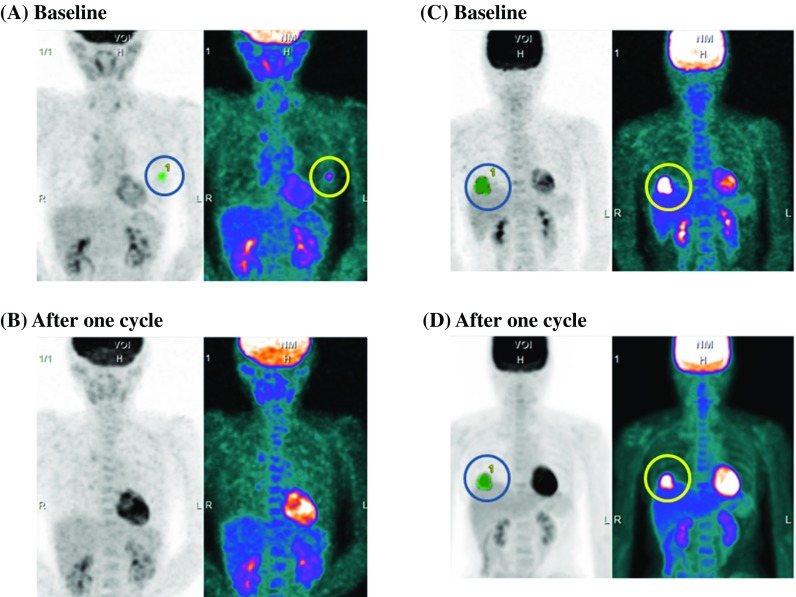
Representative cases of FDG accumulation in breast cancers at baseline and after the start of primary systemic chemotherapy. a Baseline and b after one treatment cycle in a case of breast cancer that achieved a pCR. c Baseline and d after one treatment cycle of a case of breast cancer that did not achieve a pCR. *FDG* fluorodeoxyglucose, *pCR* pathological complete response
